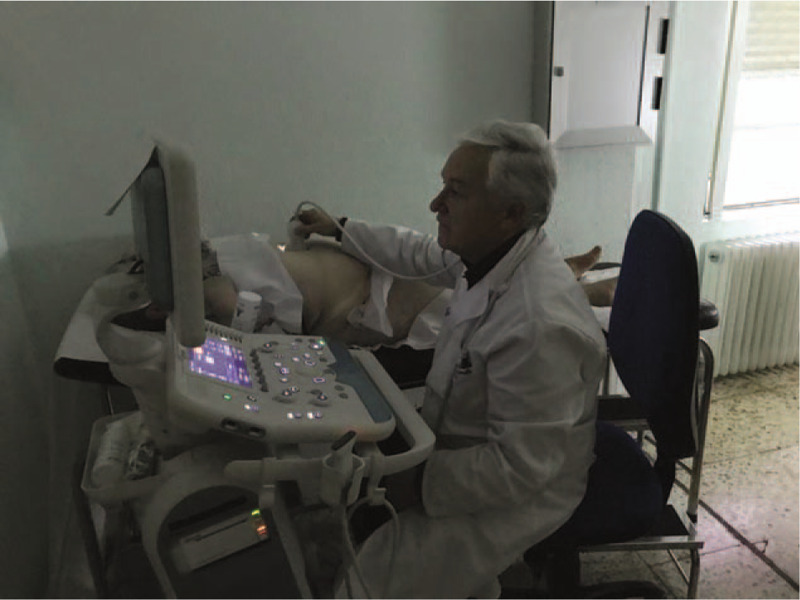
Clinical ultrasound training in the primary care clinical ultrasound classroom (AECAP).
